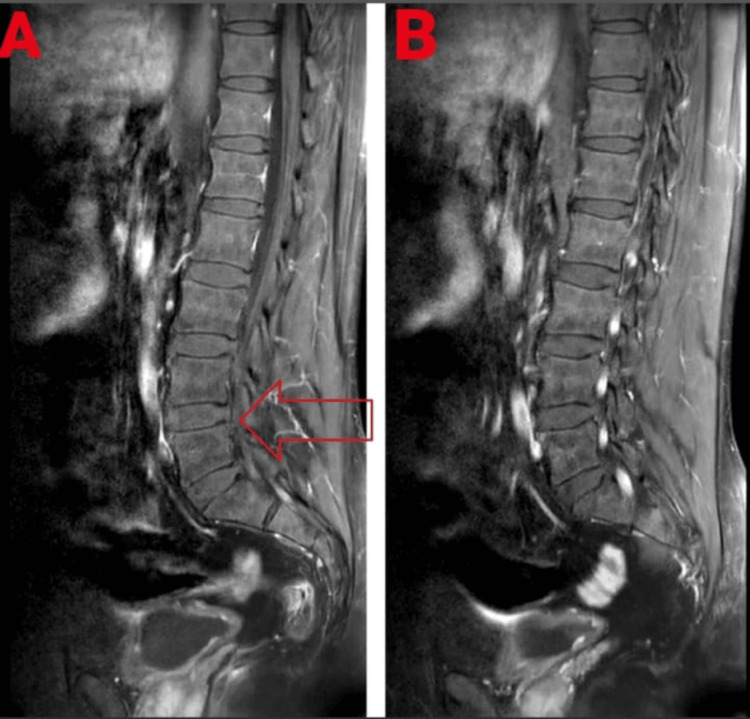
MRI scan showing (A) disc desiccation at the L4-L5 disc level, and (B) conus medularis and roots of the cauda equina depicted enhancement on contrast, raising the possibility of Guillain-Barré syndrome
